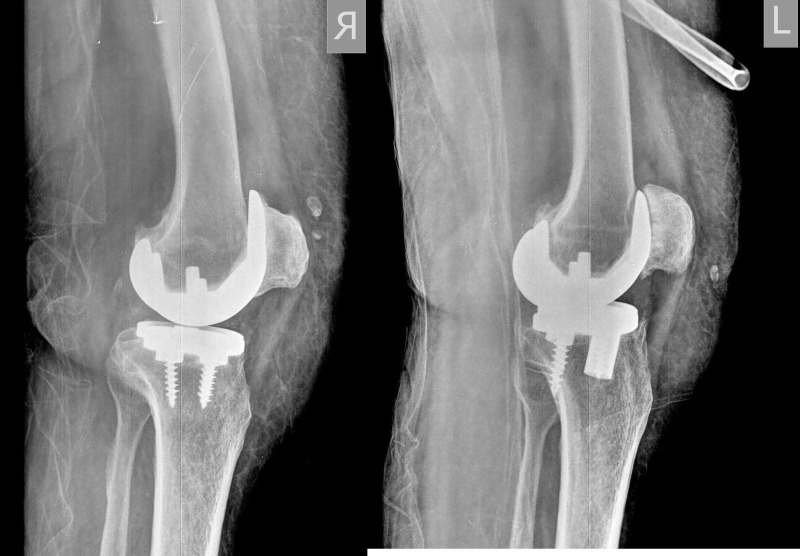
Preoperative lateral radiographs of both knees showing bilateral failed total knee arthroplasties.
